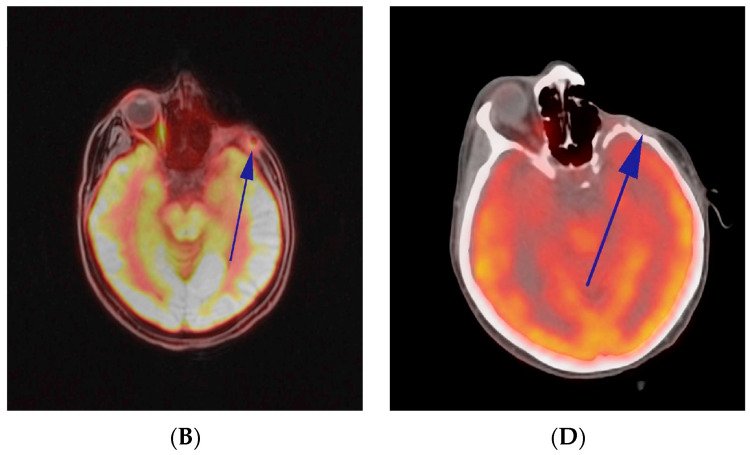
PET/CT images showing marked tumor regression and showing no pathologic FDG uptake following the treatment (pretreatment: (A,B), posttreatment: (C,D)). Blue arrows shows that zygomatic mass was eliminated upon treatment.
